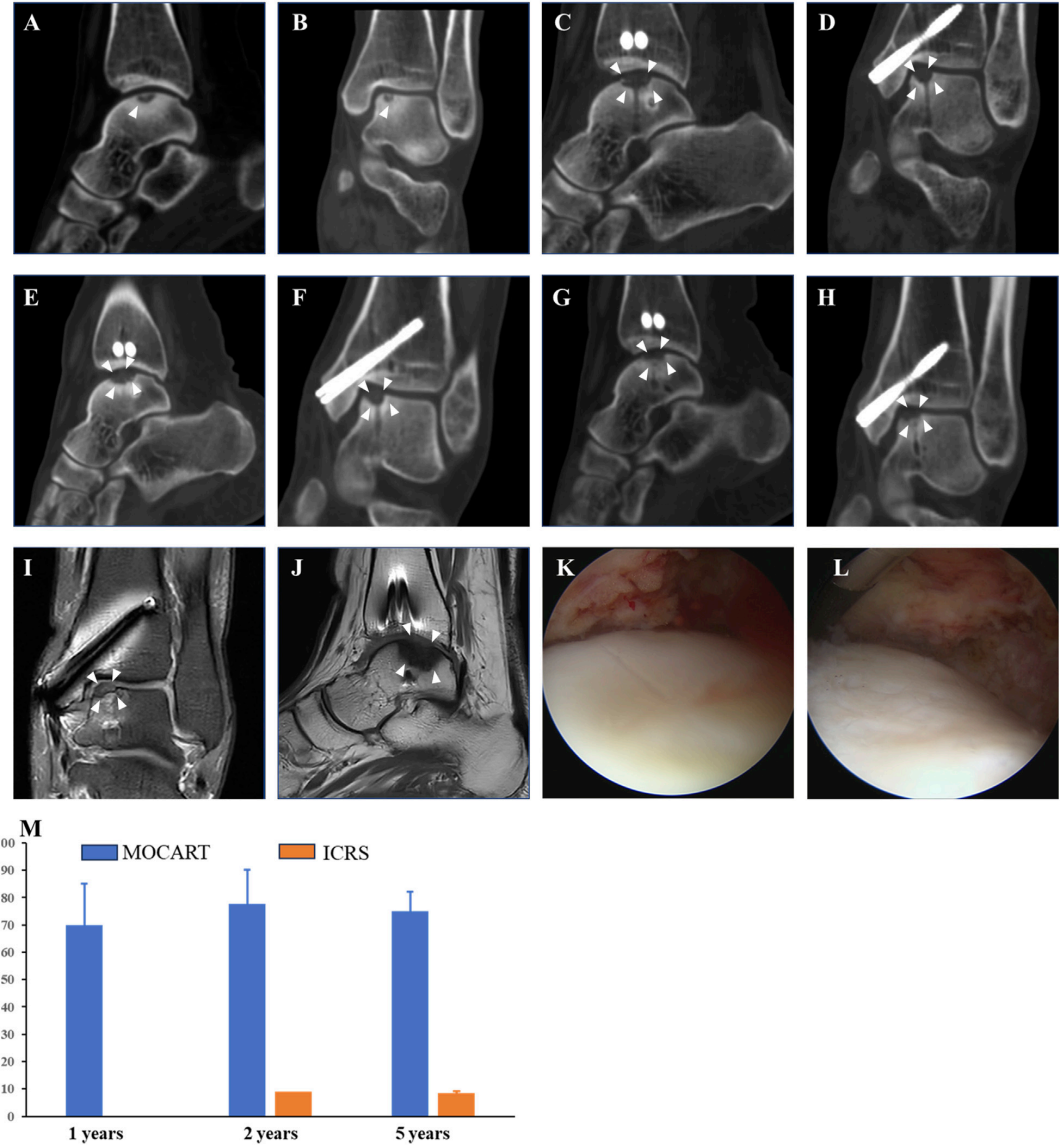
Evaluations of Imaging outcomes. 44-year-old women (patient NO.3) who were diagnosed with OLT and underwent free ACCT in 2018 with a follow-up for 5 years. The MOCART score was 70 at 1 year, 78 at 2 years, and 77 at 5 years. The ICRS score was 9 under second-look arthroscopy. (A, B) Preoperative coronal and sagittal planes of CT. (C, D) Coronal and sagittal planes of CT at 1 year after surgery. (E, F) Coronal and sagittal planes of CT at 2 years after surgery. (G, H) Coronal and sagittal planes of CT at 5 years after surgery. (I, J) Coronal and sagittal planes of MRI at 5 years after surgery. (K, L) Arthroscopy at 5 years after surgery. (M) MOCART and ICRS scores. White arrow, region of interest. *Abbreviations*: ICRS, International Cartilage Repair Society score; MOCART, Magnetic Resonance Observation of Cartilage Repair Tissue score.
